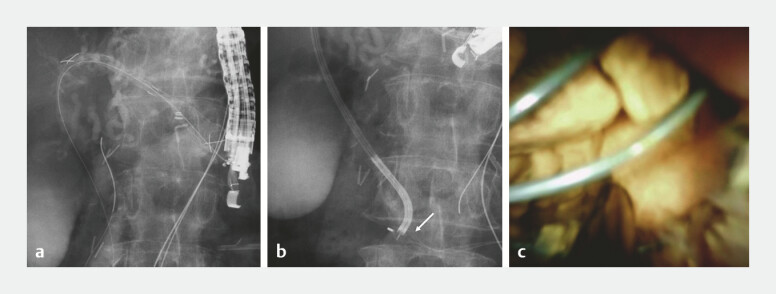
Salvage transmural lithotripsy via endoscopic ultrasound-guided hepaticogastrostomy.
